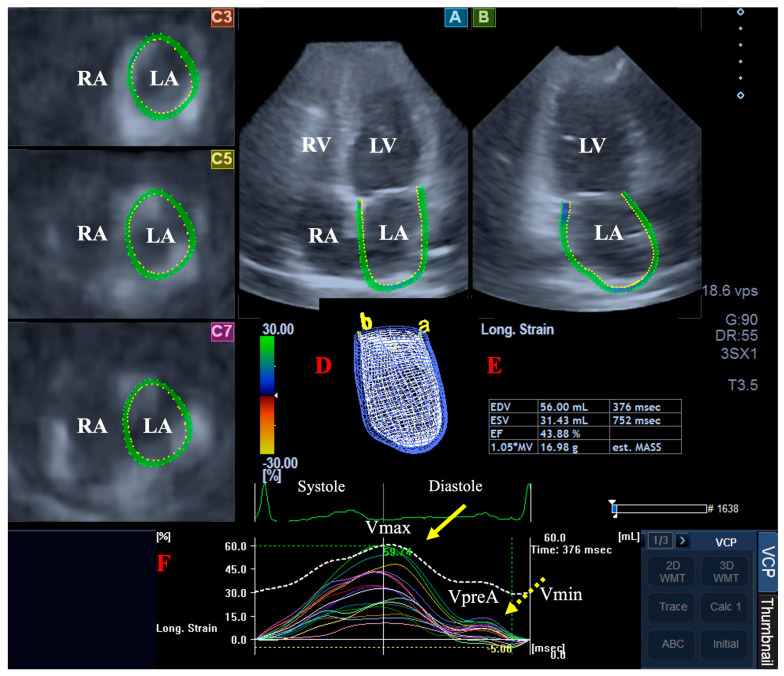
Three-dimensional (3D) speckle-tracking echocardiography-derived assessment of the left atrium (LA) in a healthy subject: apical longitudinal four-chamber (A) and two-chamber views (B) and superior (C3), midatrial (C5), and basal (C7) short-axis views are shown together with 3D cast of the LA (D) and volumetric LA parameters (E). Time–LA volume changes (dashed white line) and time–global (white line) and segmental (colored lines) longitudinal peak LA strains (yellow arrow) and LA strains at atrial contraction (dashed yellow arrow) (F) are also presented. Abbreviations. LV = left ventricle; LA = left atrium; RA = right atrium; RV = right ventricle; V_max_ = systolic maximum LA volume; V_preA_ = early diastolic pre-atrial contraction LA volume; V_min_ = late diastolic LA volume.
